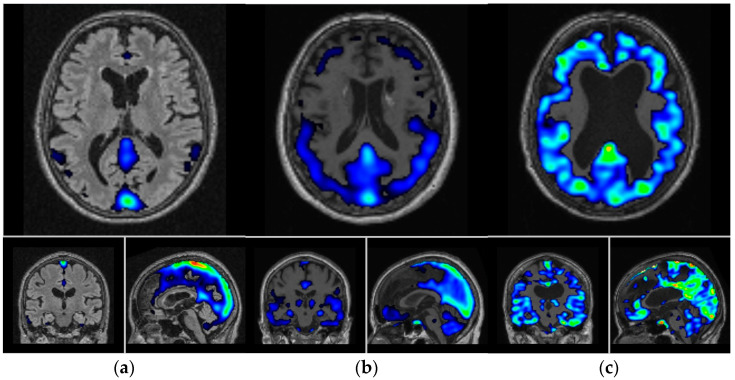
The SUV-R images of dementia patients with (a) low, (b) moderate, and (c) pronounced accumulation of [11C]PBB3. The SUV-R images overlaid on subject-specific structural MR images in the Montreal Neurological Institute (MNI) atlas space. The cerebellum was used as a reference region. Department of Nuclear Medicine. 2023. “SUV-R in C11-PBB3”. Department of Nuclear Medicine, University Hospital Ulm.
